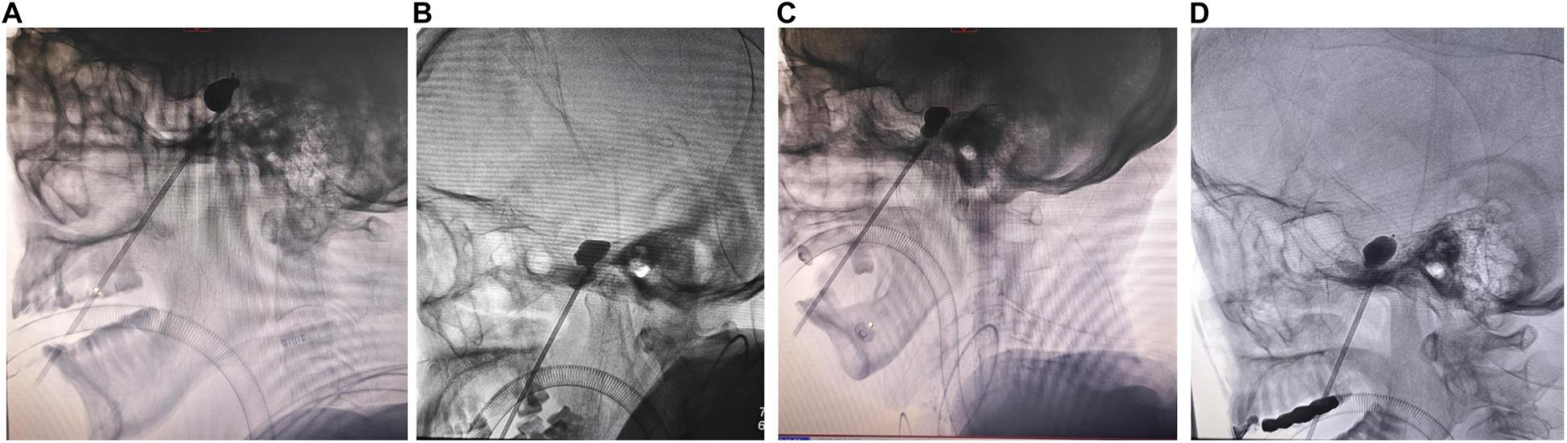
The appearance of the balloon under x-ray fluoroscopy. (A) Lateral skull radiographs confirm the ideal pear-shaped balloon. (B) Lateral skull radiographs reveal an atypical pear-shaped balloon. (C) Lateral skull radiographs indicate a dumbbell-shaped balloon. (D) Lateral skull radiographs indicate an oval-shaped balloon.
